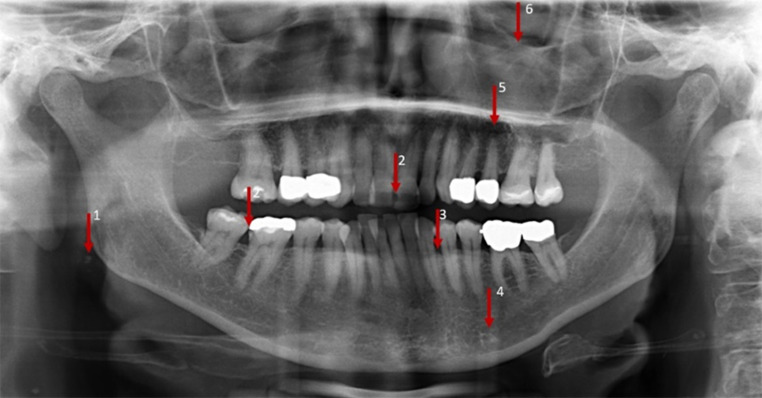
Orthopantomogram image of an adult patient with six incidental findings. Extradental incidental findings: arteriosclerosis of the external carotid artery (1), suspected periodontitis UJ/LJ (3), sclerosis of the left anterior mandible (mandibular region located apical of lower second premolar) (4), suspected apical radiolucency 25 (5), maxillary sinus opacity (6). Dental incidental findings: caries 46 and/or 21 (2). *UJ* upper jaw, *LJ* lower jaw Orthopantomogramm-Aufnahme eines erwachsenen Patienten mit 6 Nebenbefunden. Extradentale Zufallsbefunde: Arteriosklerose der Arteria carotis externa (1), Verdacht auf Parodontitis OK/UK (3), Sklerose des linken vorderen Unterkiefers (Unterkieferregion apikal des unteren zweiten Prämolaren) (4), Verdacht auf apikale Radioluzenz 25 (5), Opazität der Kieferhöhle (6). Dentale Zufallsbefunde: Karies 46 und/oder 21 (2). *OK *Oberkiefer, *UK *Unterkiefer
